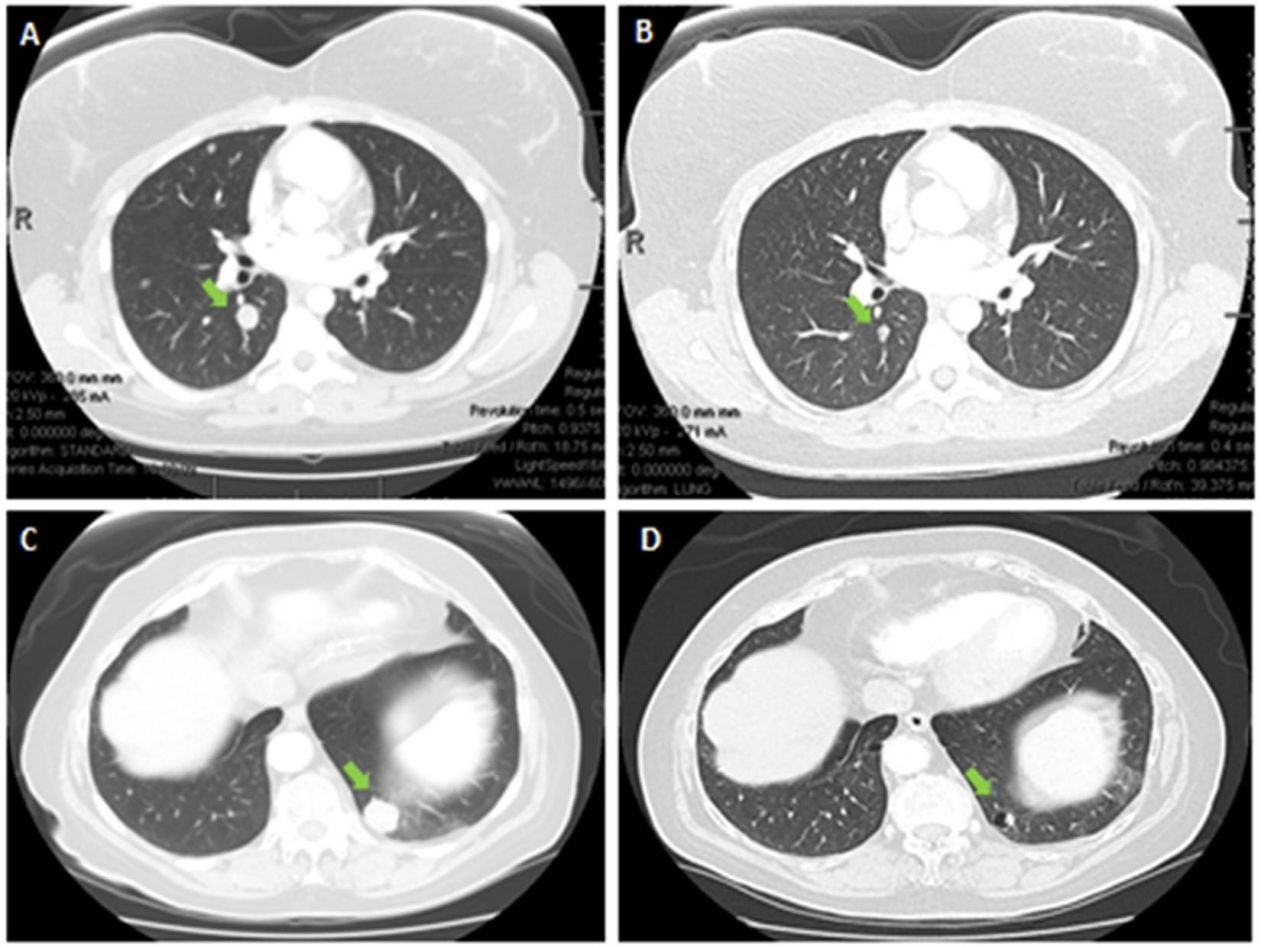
Pre- and post-treatment computed tomography scans of patients with sarcoma showing partial responses to pazopanib combinations. (A), (B) Alveolar soft part sarcoma before (A) and after (B) treatment, with 40% decrease in size of lung metastases (green arrow) per RECIST. (C,D) Angiosarcoma of the scalp metastatic to the lung before (C) and after (D) treatment, with 54% reduction in size of lung metastases per RECIST.
